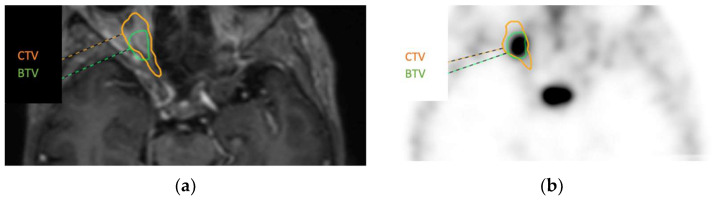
Case 3. Axial slices showing in MRI (a) and ^68^Ga-DOTATOC PET (b) the clinical target volume (CTV) and the biological target volume (BTV) of 2.5 and 1/1 cm^3^, respectively.
